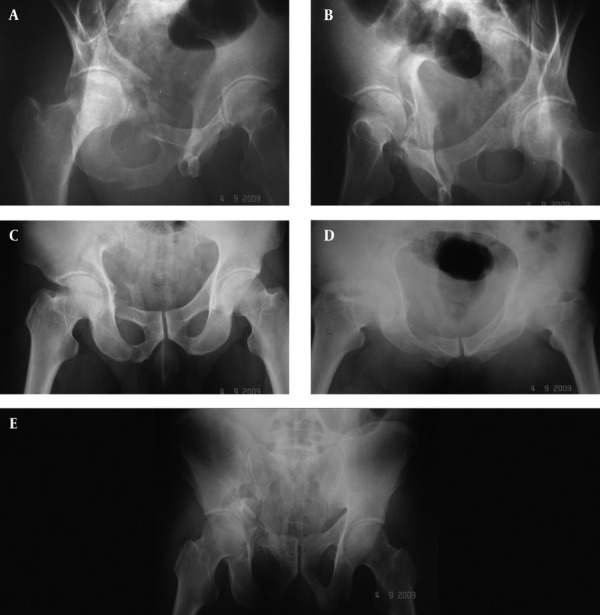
Preoperative Radiographs. A) Obturator oblique view indicates the fracture in the anterior acetabular column and superior and inferior rami of the pubis in the right hip; B) Iliac oblique view indicates the fracture line in the weight bearing dome extending to the SI joint without involving the posterior column; C) Anteroposterior view indicates the fracture line in superior articular surface extending to the SI joint; D) Inlet view indicates the fracture in the pubic rami; E) Outlet view indicates the posterior iliac wing and pubic rami fractures.
